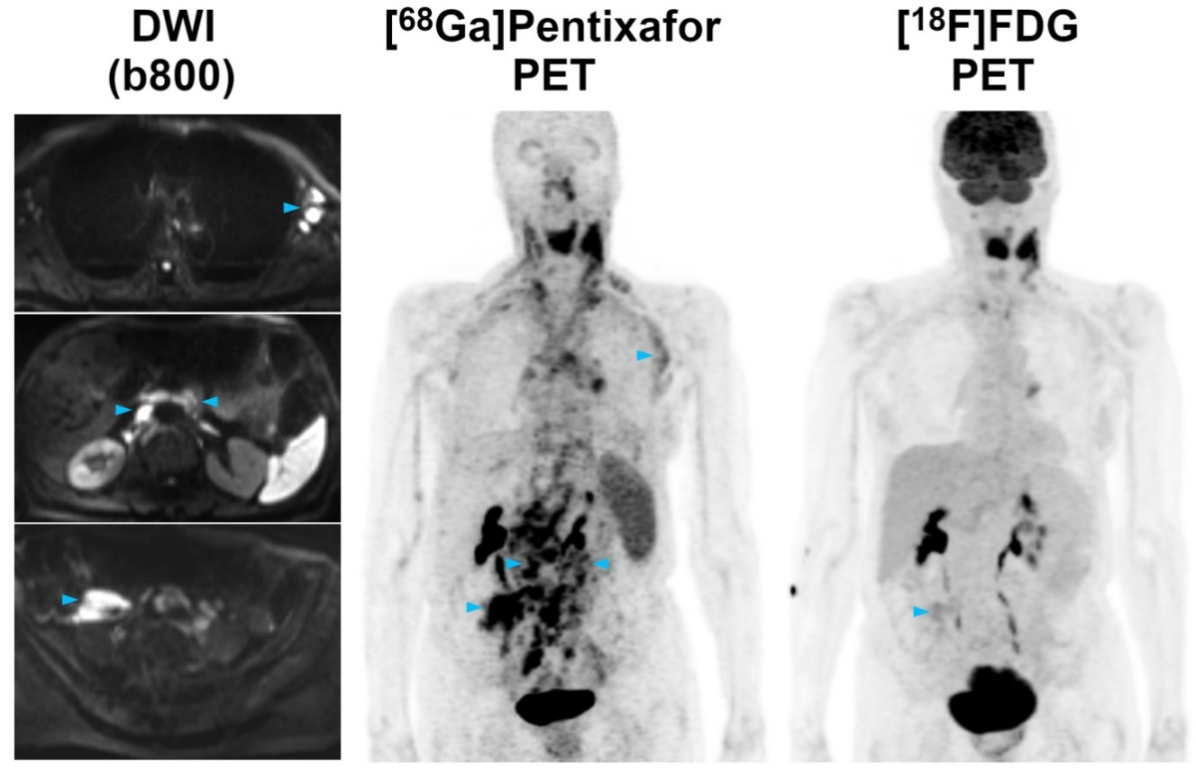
Pre-therapeutic [^68^Ga]Pentixafor-PET/MRI and [^18^F]FDG-PET/MRI of an 82-year-old female MCL patient. [^68^Ga]Pentixafor-PET detects considerably more nodal lymphoma manifestations than [^18^F]FDG-PET, and stronger tracer uptake in several lesions/regions. All lymph nodes with focal increased [^68^Ga]Pentixafor uptake corresponded to clearly enlarged lymph nodes on diffusion-weighted MRI (DWI). Representative examples of such [^68^Ga]Pentixafor-PET-positive lesions with no or low [^18^F]FDG uptake, but obvious lymphadenopathy on DWI, are marked by blue arrowheads (left axially, retroperitoneal/periaortic, and right pelvic regions).
